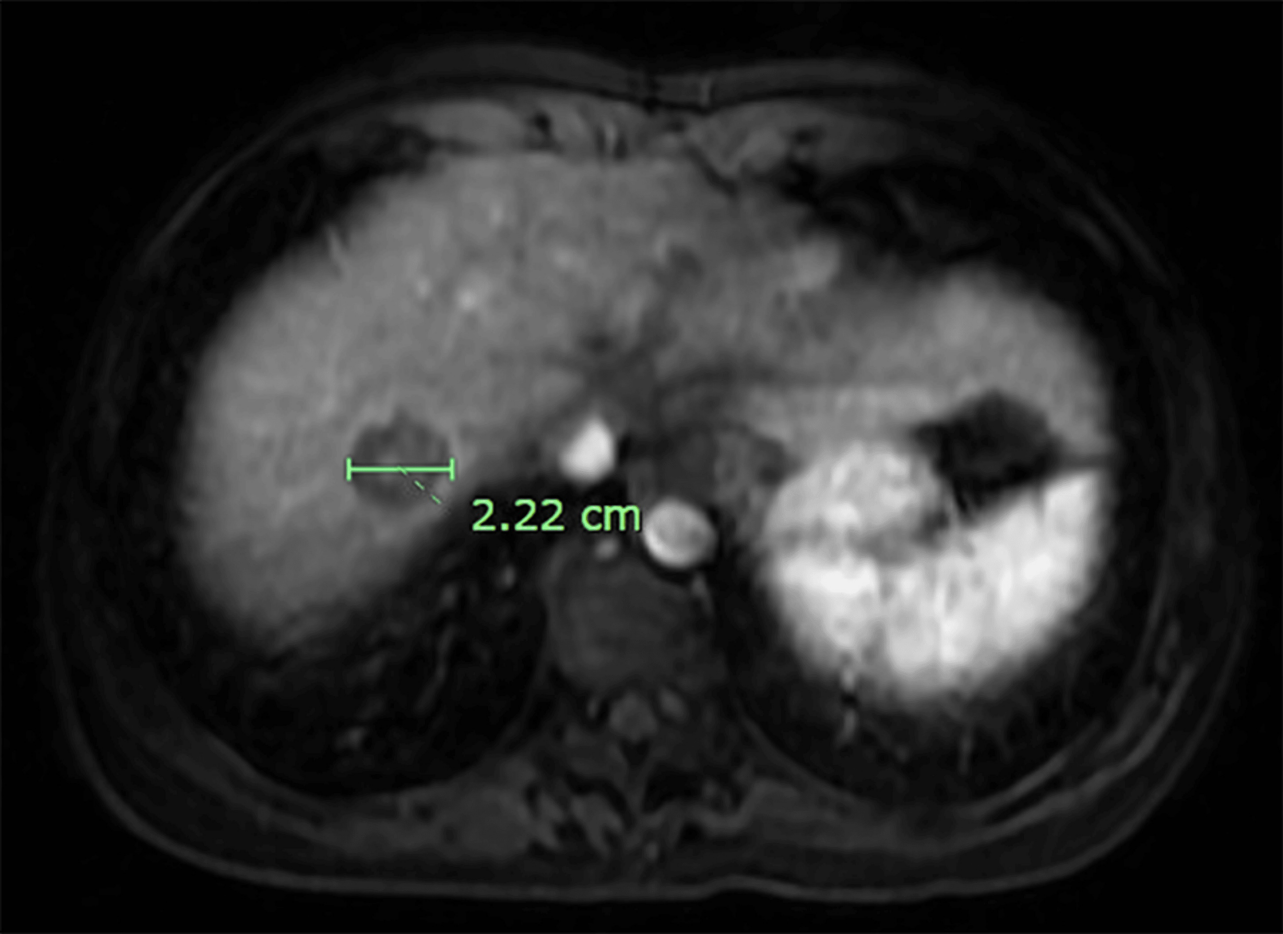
Magnetic resonance imaging (MRI) of the abdomen with and without intravenous contrast. The green line shows the maximum diameter of the liver nodule detected in hepatic segment 8.
